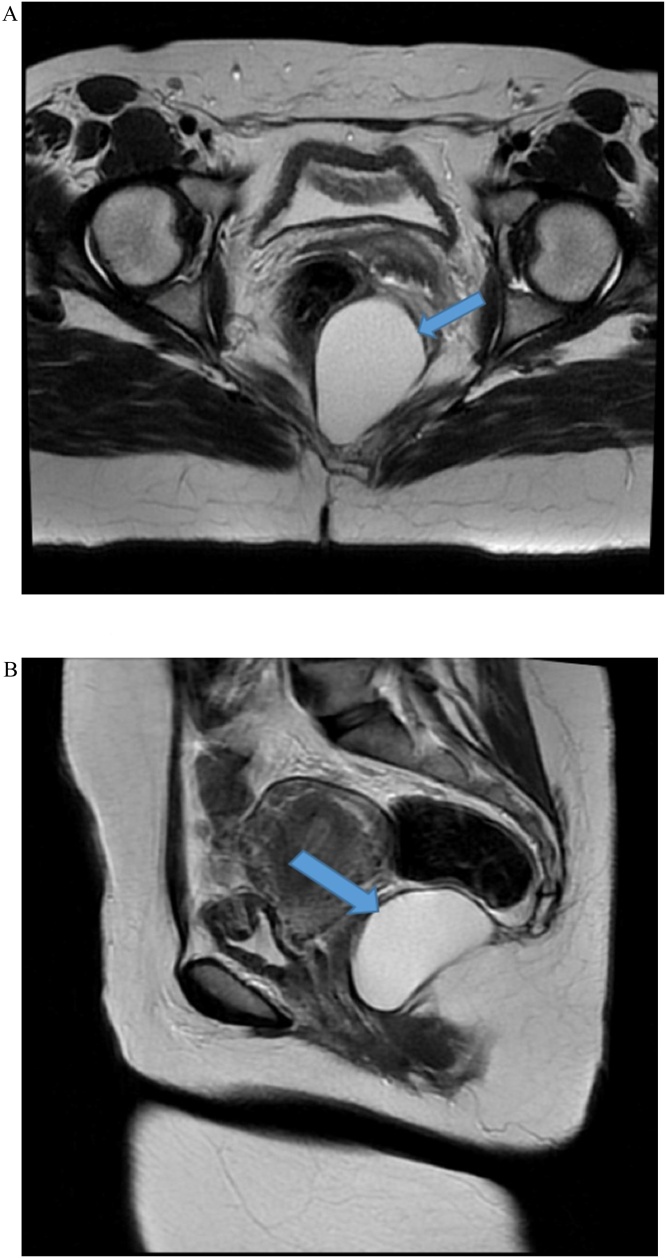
A: MRI of abdomen confirmed a well-defined homogeneous at the lower pelvis, retrorectal in location, approaching the level of the anal canal. Anterior displacement of the rectum was seen with no signs of rectal wall signal alteration to suggest infiltration. B: Sagittal view of the same image.
